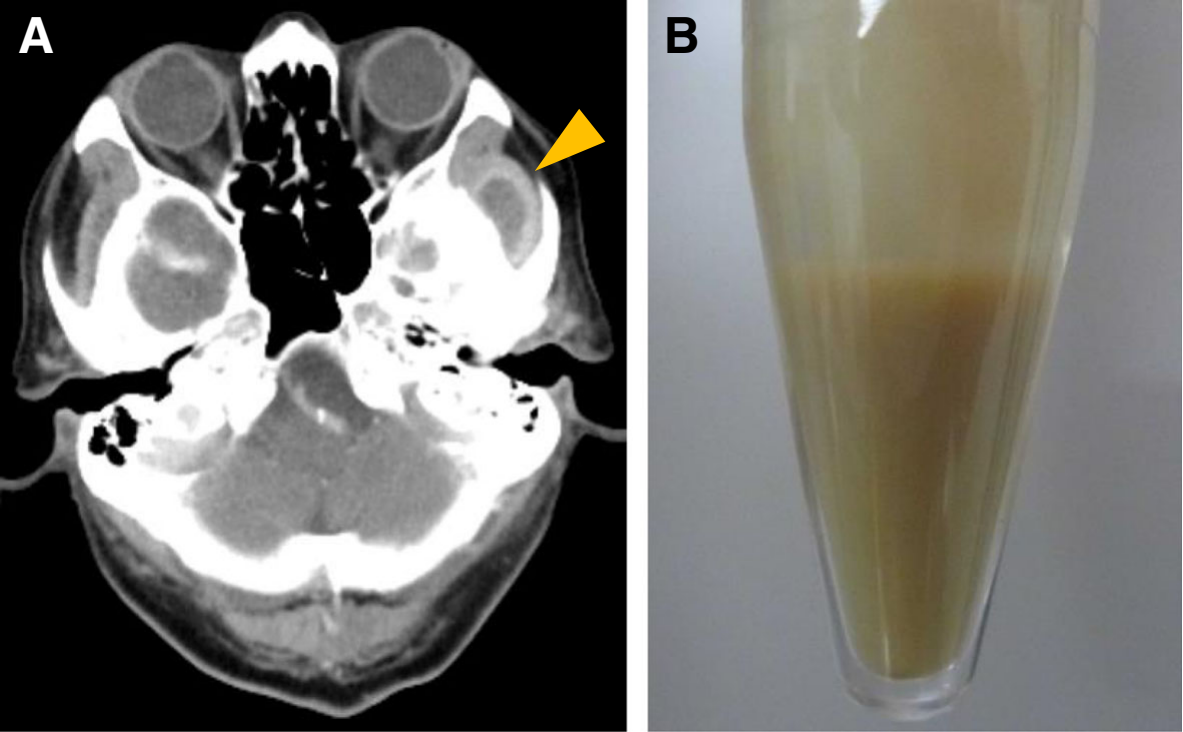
Images of infratemporal fossa abscess. a Contrast-enhanced head CT scan. Arrowheads indicates infratemporal fossa abscess. b Photograph of aspirated abscess
